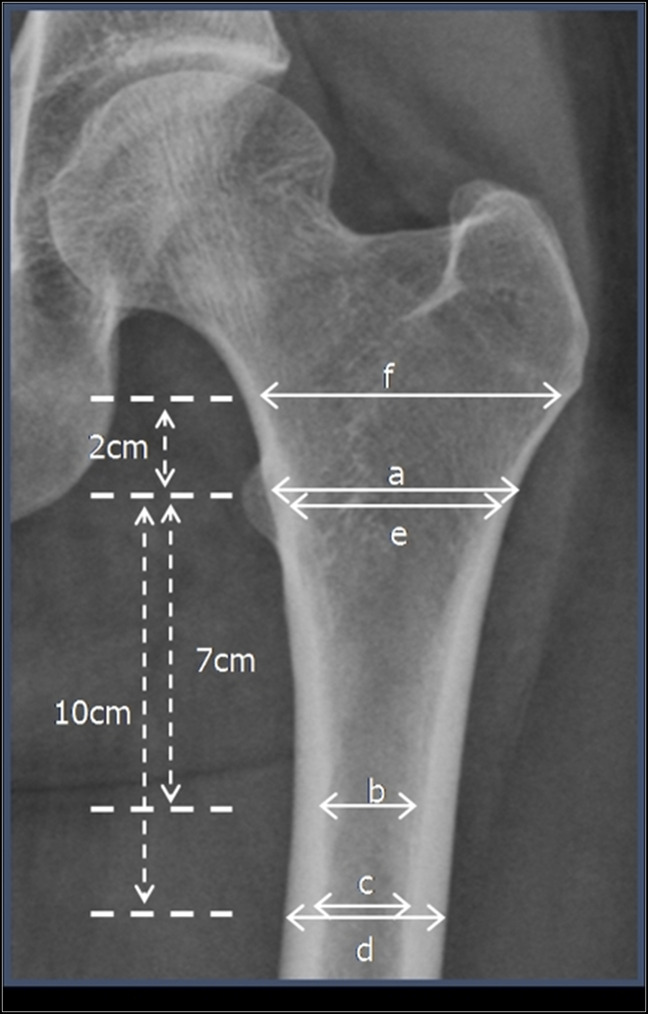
Radiograph showing analysis to ascertain the proximal femoral morphology. Reprinted from Early Post-operative Periprosthetic Femur Fracture in the Presence of a Non-cemented Tapered Wedge Femoral Stem, Cooper HJ et al, 2010, by the *HSS Journal: the Musculoskeletal Journal of Hospital for Special Surgery*. cortical index = a/b, canal cone ratio = c/d, canal to calcar ratio = c/e, canal-flare index = f/c
